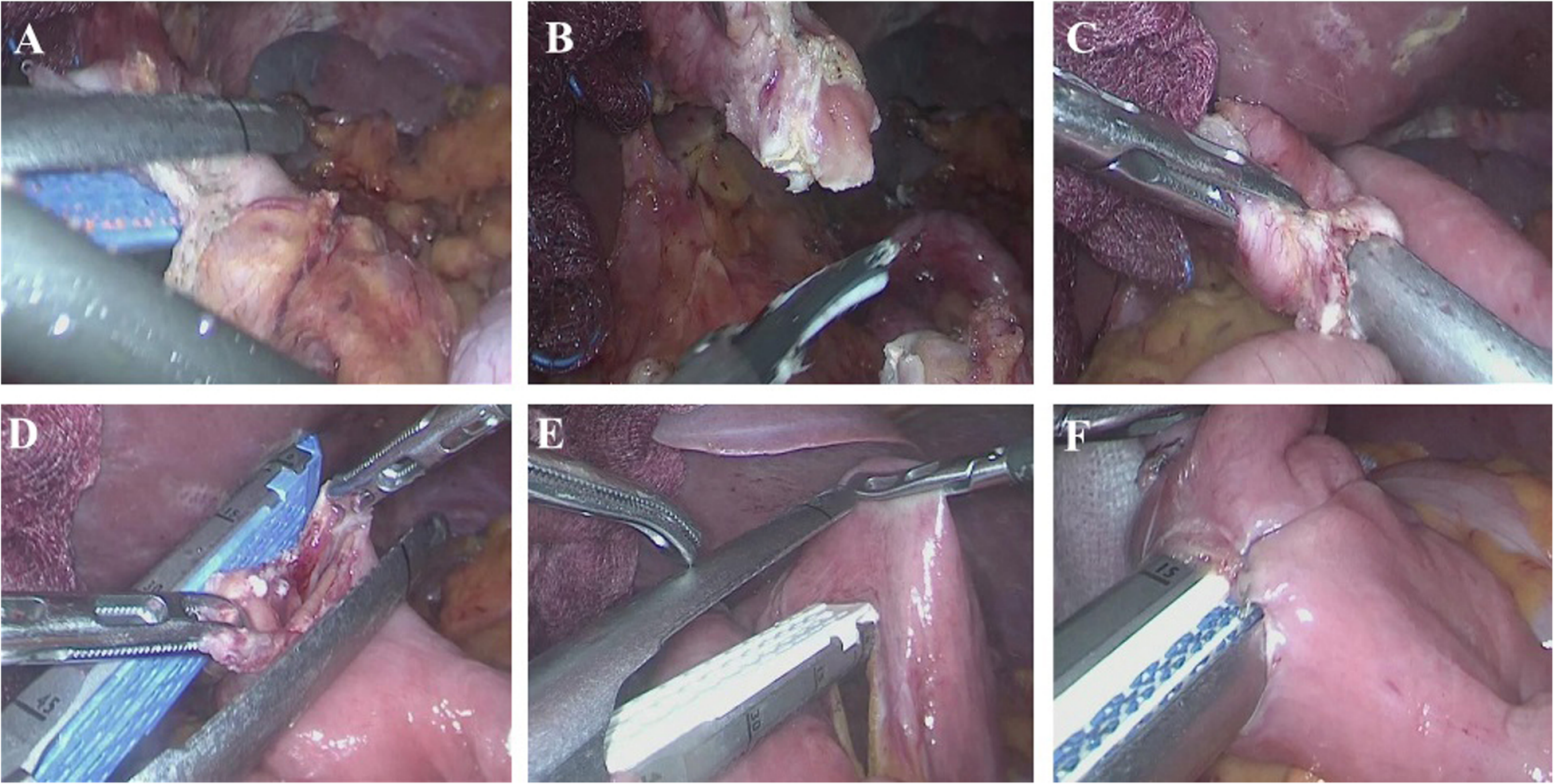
Esophagojejunostomy using the linear stapling technique. a Partial transection of the esophagus using a linear stapler. b Complete transection of the esophagus using an ultrasonic device. c Intracoporeal anastomosis using the overlap method. d Closure of the entry hole. e Jejunum transection without mesenteric division using a linear stapler. f Jejunojejunostomy 50 cm distal to the esophagojejunostomy
